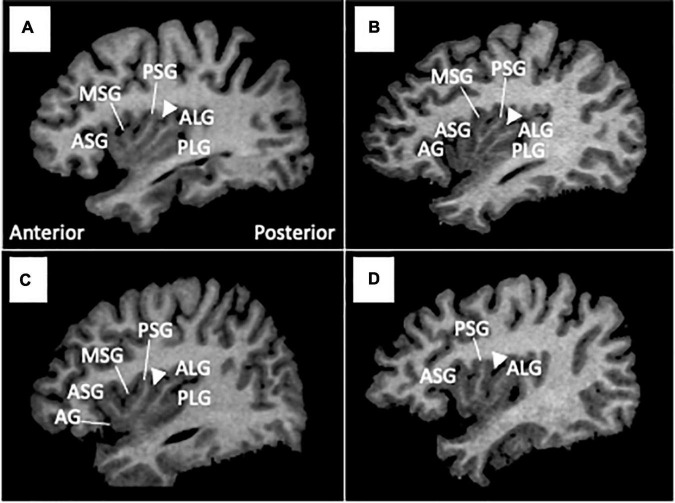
Insular gross anatomical variations in sample MR images in sagittal views. Coronal and axial views were simultaneously referred to in assessments of gyral development. Arrowheads indicate the location of the central insular sulcus, which subdivides the short (anterior) and long (posterior) insular cortices. The ASG, PSG, and ALG were well-developed in all participants in this study, while the AG and MSG were absent [subject (D)], underdeveloped [subject (C)], or well-developed [subject (B)]. The PLG was present in most subjects (A–C), but was not observed in subject (D). AG, accessory gyrus; ALG, anterior long gyrus; ASG, anterior short gyrus; MSG, middle short gyrus, PLS, posterior long gyrus; PSG, posterior short gyrus.
